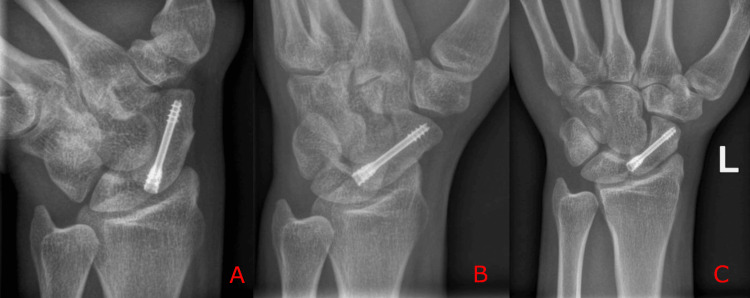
Postoperative six-month wrist radiographs demonstrating healed scaphoid fracture (A–C) PA in ulnar deviation, oblique and posteroanterior radiographs showing complete fracture healing with the headless compression screw in situ.
